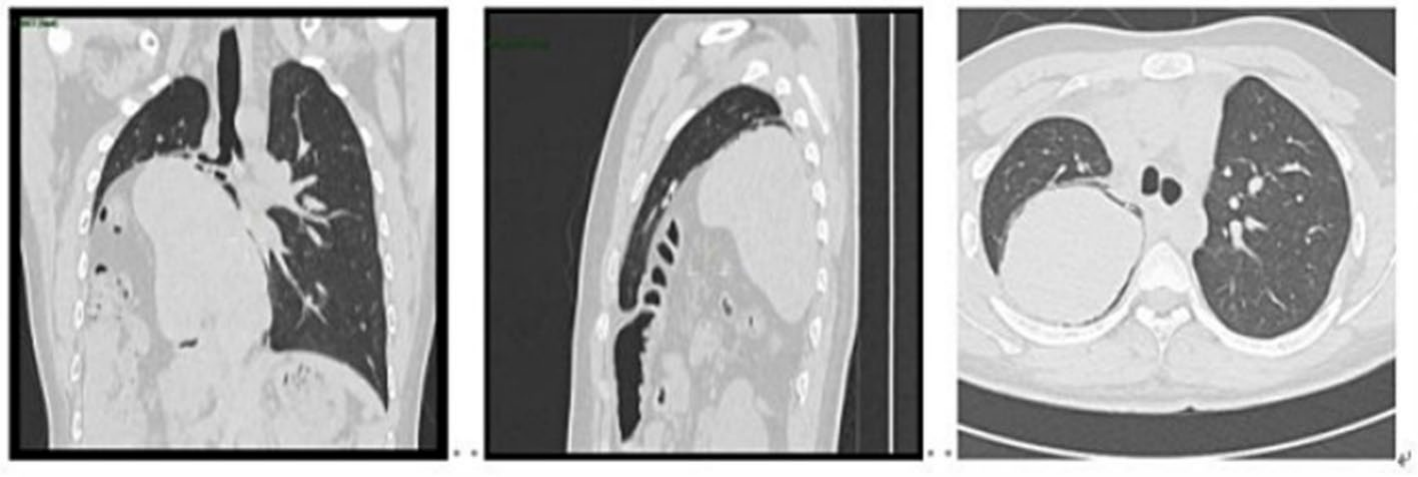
Preoperative chest CT showing right-sided diaphragmatic hernia with intrathoracic displacement of the liver and mediastinal shift.
